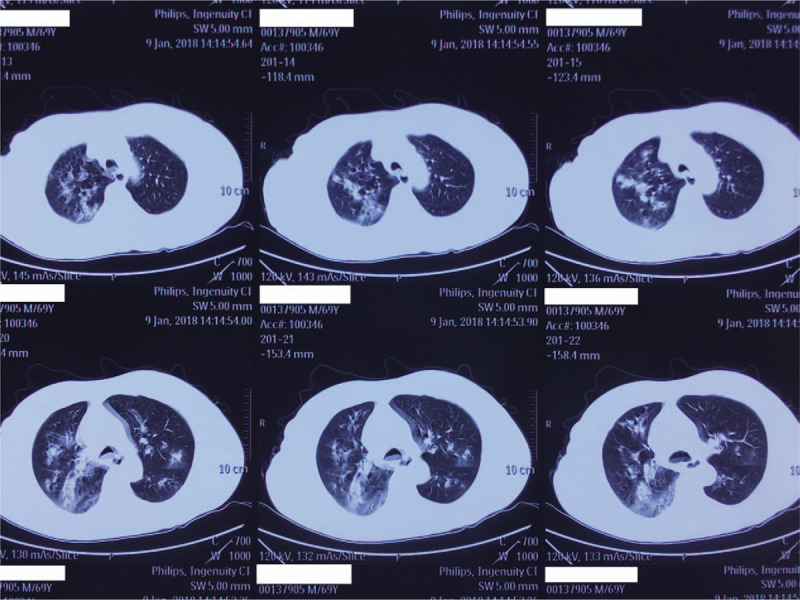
A computed tomography scan of the chest performed on January 9, 2018.
